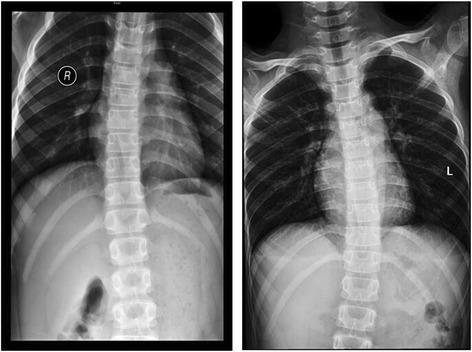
The spine radiograph showing the litter patient has scoliosis
